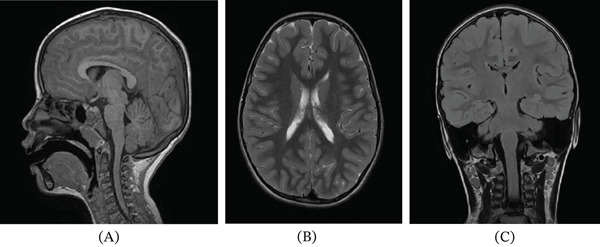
Brain MRI of index case. (A) Sagittal T1‐weighted, (B) axial T2‐weighted, and (C) coronal T2‐weighted images obtained at age 5. Brain structures appeared normal with no clear abnormalities.
